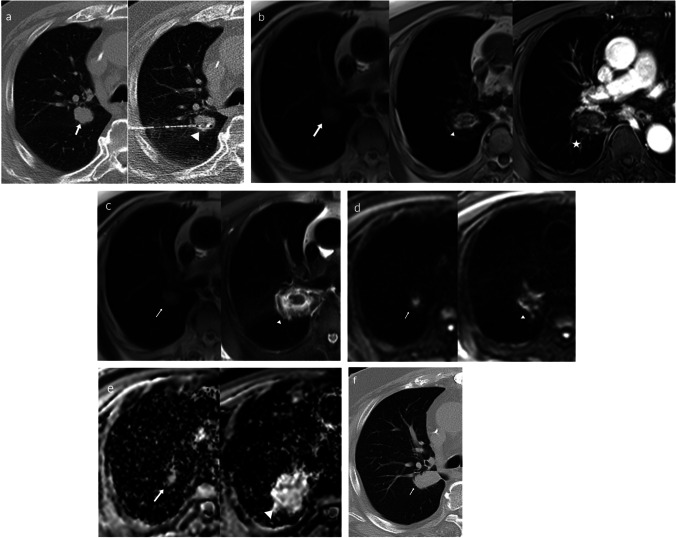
A 75-year-old male patient with metachronous pulmonary metastasis from colorectal cancer. a Combined CT images showing the pre-ablation right perihilar metastatic lesion (left-image arrow) and CT image during MWA (arrowhead). b Combined MRI images in T1WI pre-ablation (left image), T1WI 24 h post-ablation (middle image), and T1WI with contrast 24 h post-ablation (right image) displaying low signal intensity before and after ablation with marginal contrast enhancement after ablation. c Combined MRI images in T2WI pre-ablation (left image) and T2WI 24 h post-ablation (right image) displaying high signal before ablation and central low signal intensity with marginal high signal after ablation. d DWI pre-ablation (left image) and DWI 24 h post-ablation (right image) displaying high signal intensity before ablation with reduction of the signal intensity after ablation. e ADC map pre-ablation (left image) and ADC map 24 h post-ablation (right image). f In the CT follow-up after 6 months, the lesion shows a fibrotic band with no contrast enhancement denoting local tumor response
